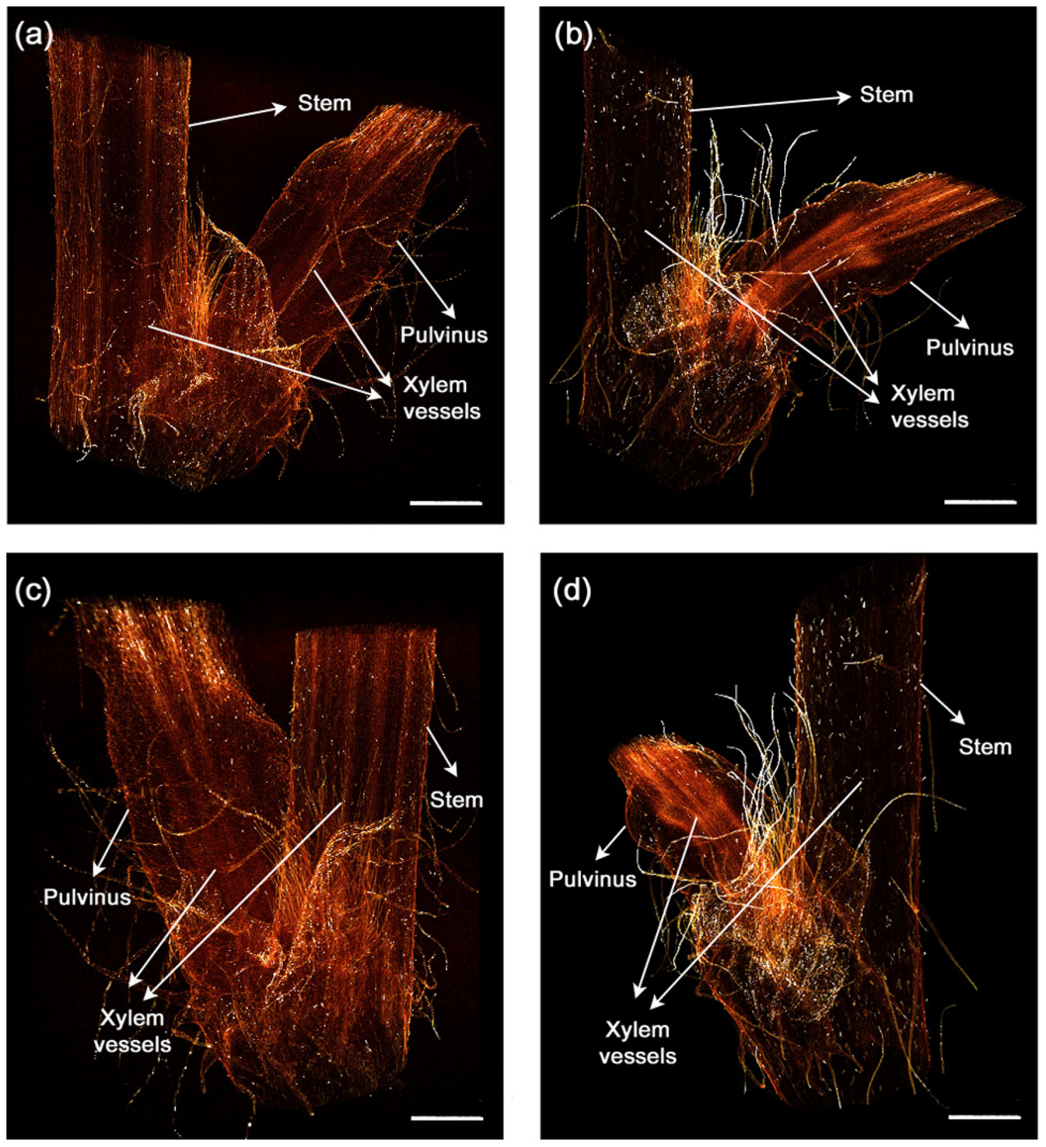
3D flexible structures of the straight and bending pulvinus. (a–d) X-ray tomograms show the internal morphological structure of the two different pulvini. 3D reconstructed images of the straight (a, c) and the bending (b, d) pulvini. The pulvinus reconstructed in a flank view (a, b) and a 130° rotated view around the vertical axis (c, d). The xylem vessels inside the pulvinus are straight or bent depending on the morphological condition. Scale bar, 2 mm.
